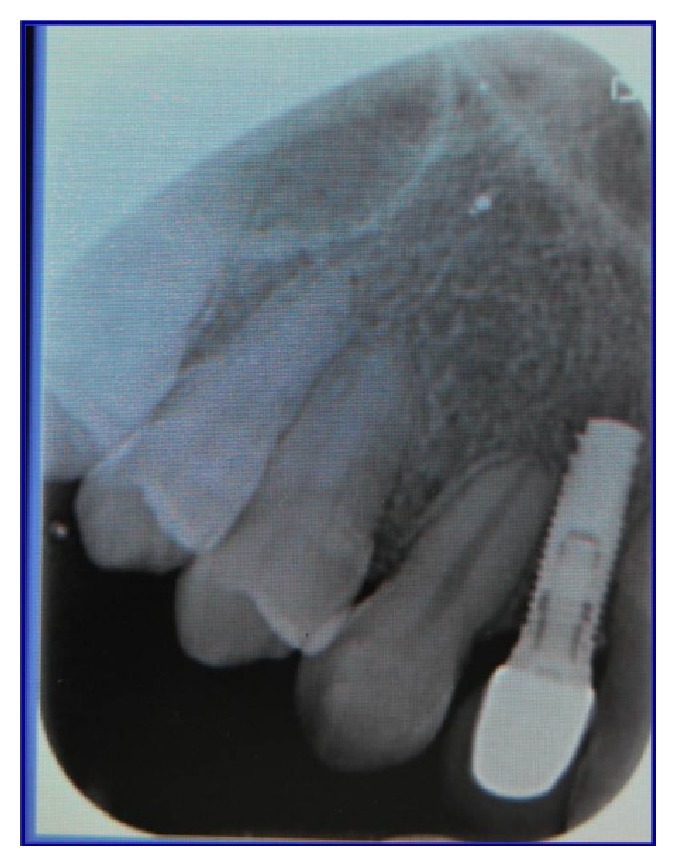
Radiographic control after one year in site to the left.
